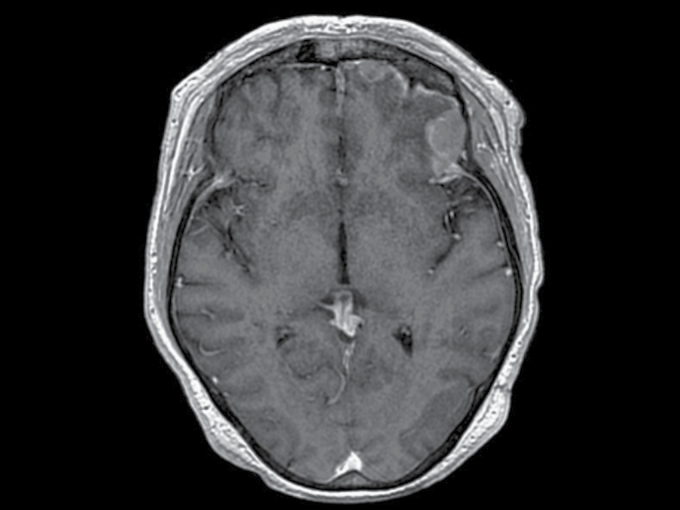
An MRI demonstrates nodular leptomeningeal recurrences (2 nodules) at the left frontal lobe adjacent to the surgical cavity in a 62-year-old non-small cell lung cancer patient receiving brain metastasis resection and cavity stereotactic radiosurgery 2 months ago.
